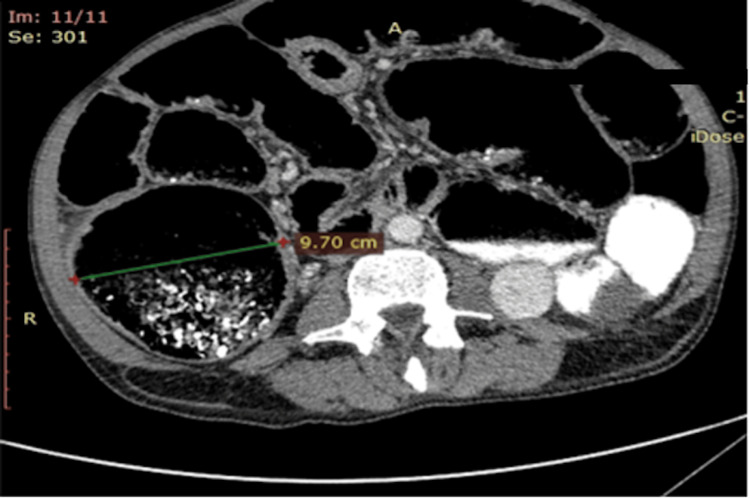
Distended cecum is shown in preoperative CT imaging in a case where cecostomy was preferred
